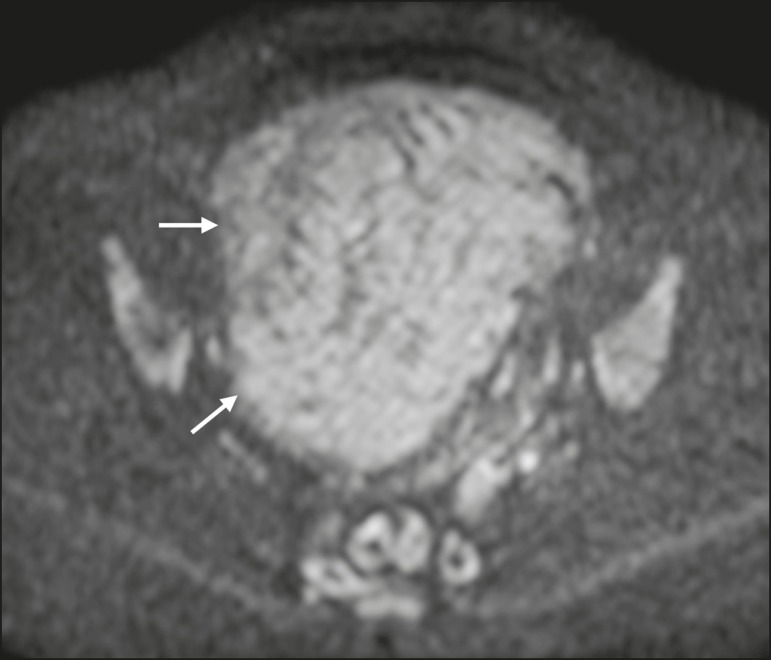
MRI of a female patient with cellular leiomyoma. Axial DWI at b = 1000
